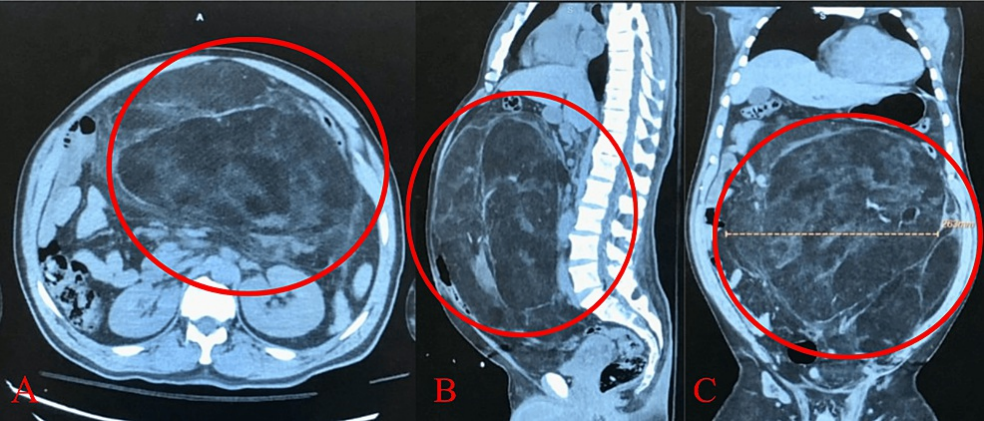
Initial computed tomography imaging without contrast in the emergency department results showed a 32 cm incidental lipomatous lesion of the left hemiabdomen with mass effect on the bowel and with mild soft tissue compartment in the axial (red circle in A), sagittal (red circle in B), and coronal planes (red circle in C).
